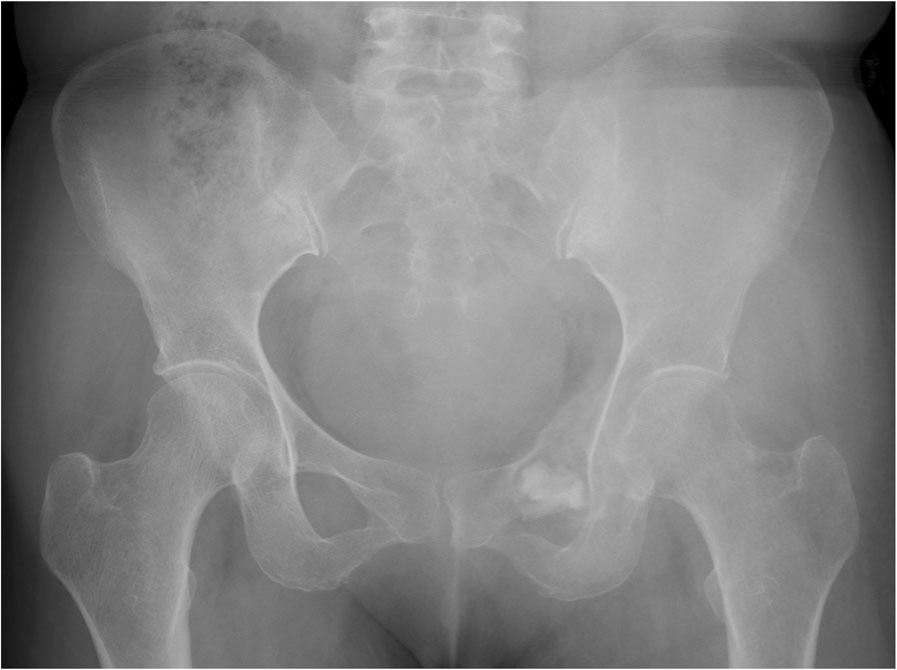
Pelvic plain radiography in a patient with an osteosarcoma (after biopsy) involving the anterior column of left acetabulum, ischium, pubis, and ilium
